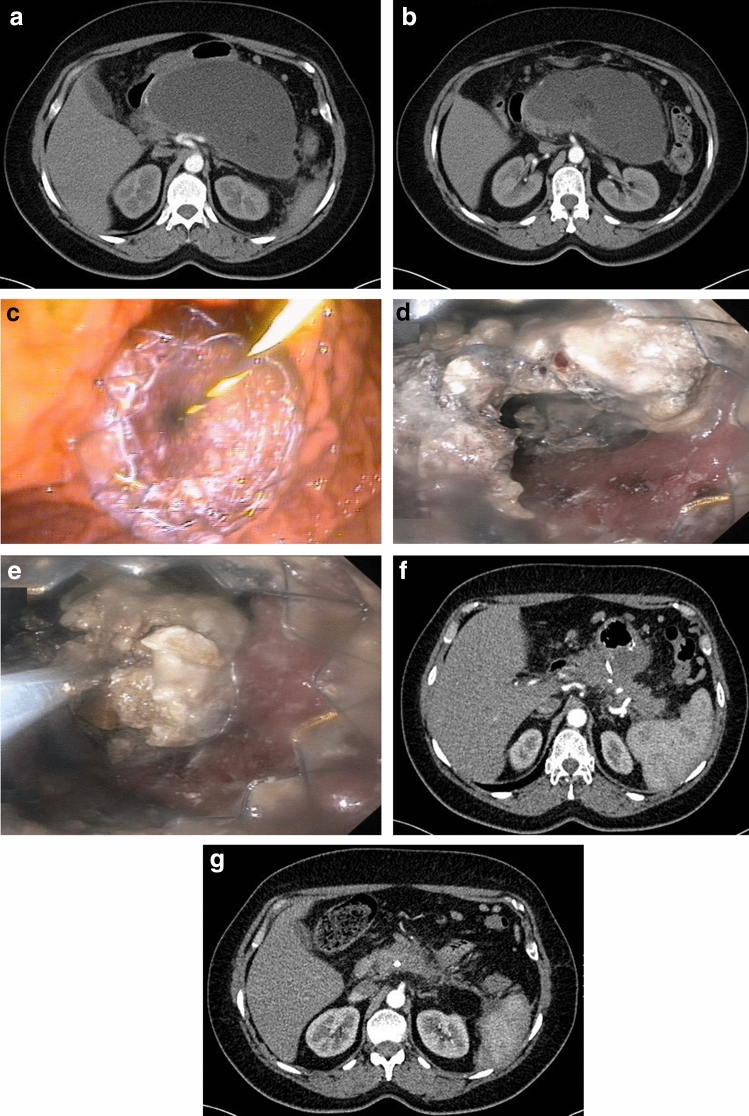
a–g Endoscopic treatment of walled-off pancreatic necrosis. A 62-year-old female patient qualified for drainage of infected walled-off pancreatic necrosis on day 48 of acute necrotizing pancreatitis (a, b). Transmural endoscopic drainage (c) and numerous direct endoscopic necrosectomy procedures were performed (d, e). On control contrast-enhanced abdominal and pelvic computed tomography (f, g) after 12 months since the endoscopic treatment, a complete regression of necrotic collections was noted.
